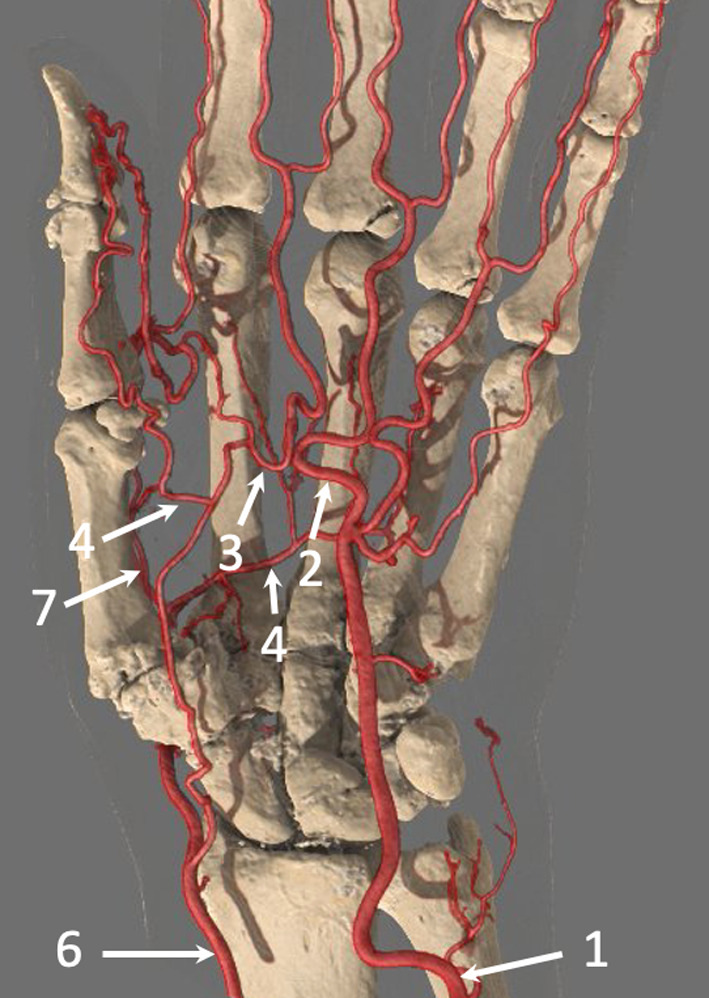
SHR-CTA of the hand (with GI) in a 69-year-old woman that shows the complex arterial network of the hand with a Type A incomplete SPA. The UA (1) feeds the ulnar side of the SPA (2), while the superficial branch of the RA feeds the radial side of the SPA (3). The SPA gives off branches into the first web space, which supply the radial side of the index finger and the thumb (4). The DPA (5) is constituted mainly from the RA (6), which forms an anastomosis with the deep branch of the UA. It generates the FPMA (7). Note the close relationship between the UA and the hamulus. Note also carpal degenerative changes and joint calcifications due to calcium pyrophosphate deposition disease
